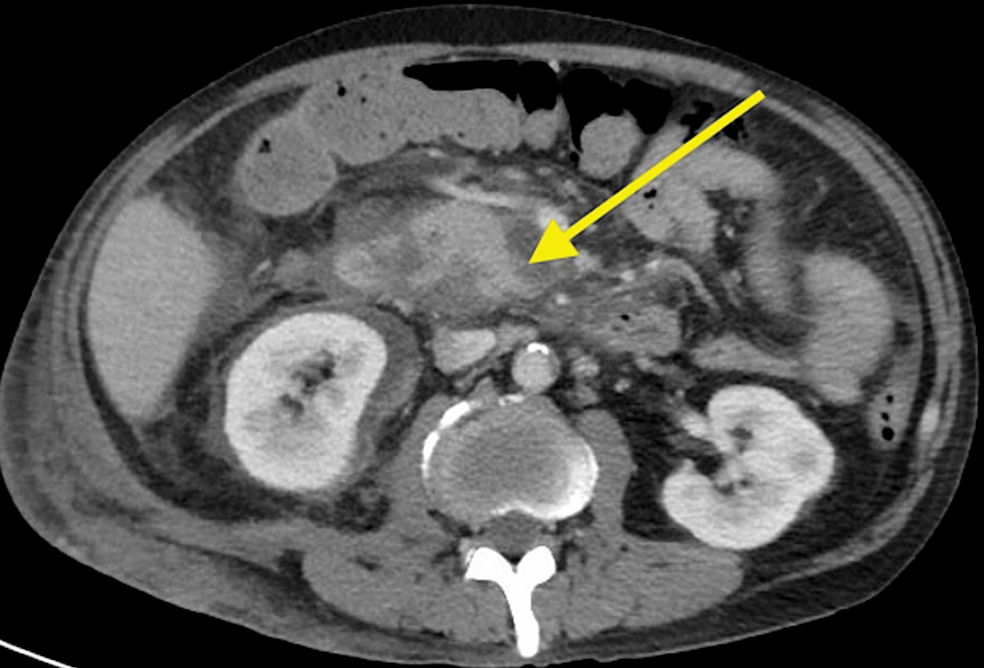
CT of the abdomen and pelvis The yellow arrow depicts the patient’s necrotizing pancreatitis and peripancreatic fluid collections found on CT imaging
